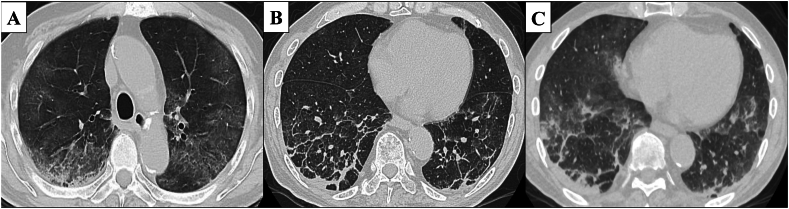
HRCT on admission Chest HRCT image on admission. (A) Diffuse ground glass opacities predominantly in the upper lobes. (B) Infiltration shadows were seen in the bilateral lower lobes. (C) Air trapping was observed in expiratory CT.
